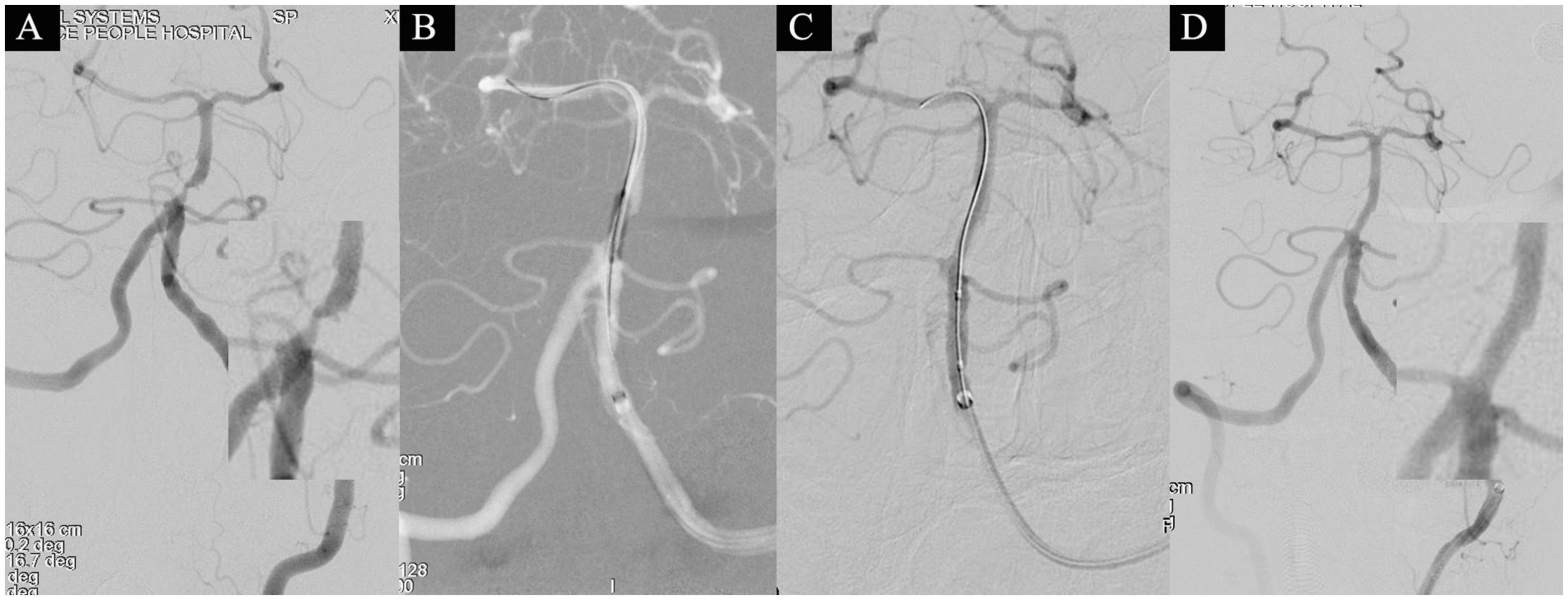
A 58-year-old male presented with sudden-onset right-sided weakness for 12 days and was admitted on November 4, 2021. Head MRI revealed a left pontine infarction, with an NIHSS score of 1 point at admission (attributed to dysarthria). On November 8th (after 16 days of stroke onset), the patient underwent a comprehensive basilar artery angioplasty procedure. Angiography identified severe stenosis in the basilar artery, characterized by a distal lumen of 2.9 mm, the narrowest segment measuring 0.4 mm, and an overall stenosis degree of 86.2% (A). Subsequent dilations were conducted using 1.5 × 15 mm and 2.5 × 15 mm balloons (B,C). Approximately 10% residual stenosis remained. Following a 15-min observation period, a subsequent angiogram exhibited no significant restenosis (D), resulting in the attainment of eTICI grade 3.
